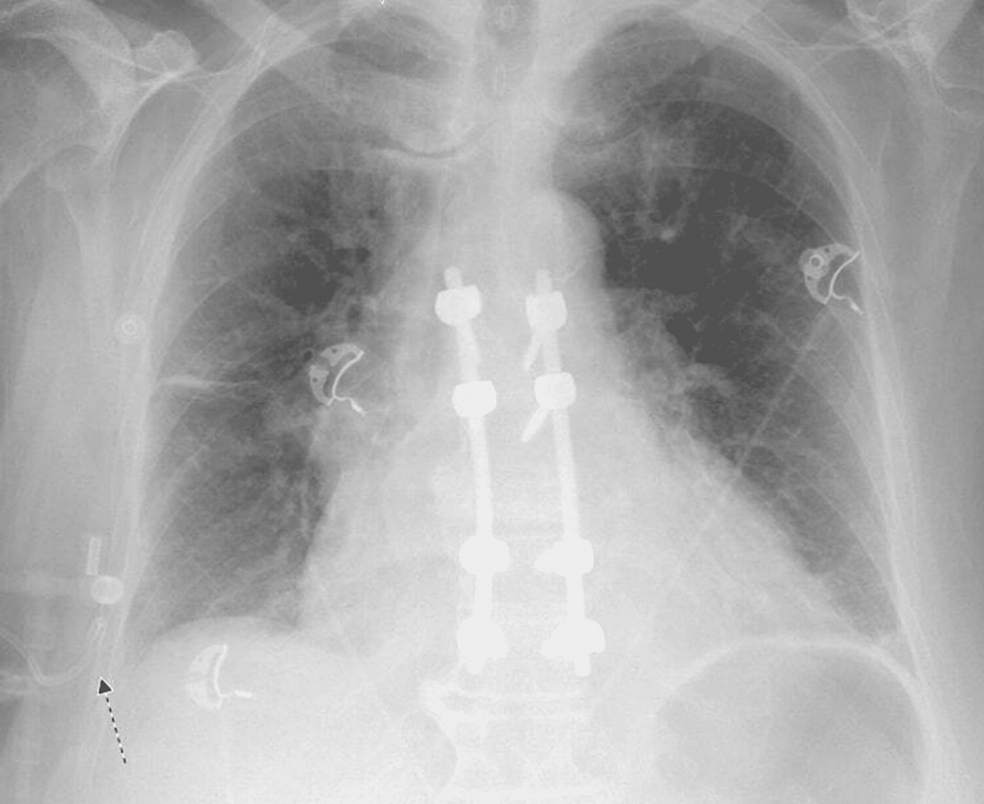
X-ray of the chest after insertion of the pleural catheter (arrow). The figure was generated entirely for this publication and gained agreement from the patient to post it.
